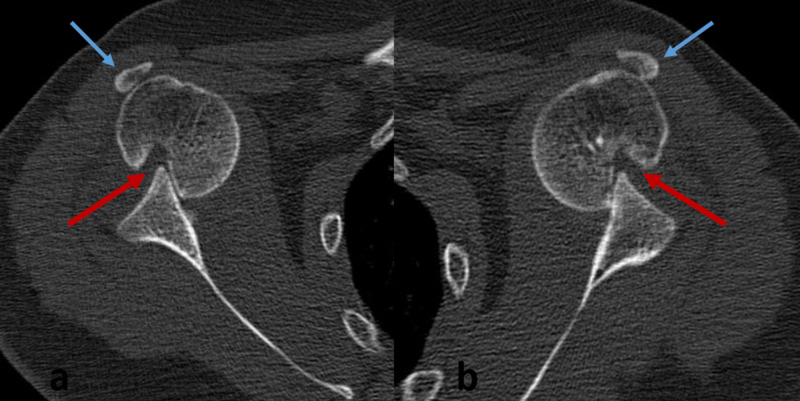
(a, b) CT Scan axial cuts of both shoulders showing bilateral anterior dislocation, with the humeral head locked over the anterior glenoid rim, Hill-Sachs lesion of approximately 20% of the humeral head (red arrow), and fracture of the coracoid tip displaced to the lateral aspect of the greater tuberosity (blue arrow)
